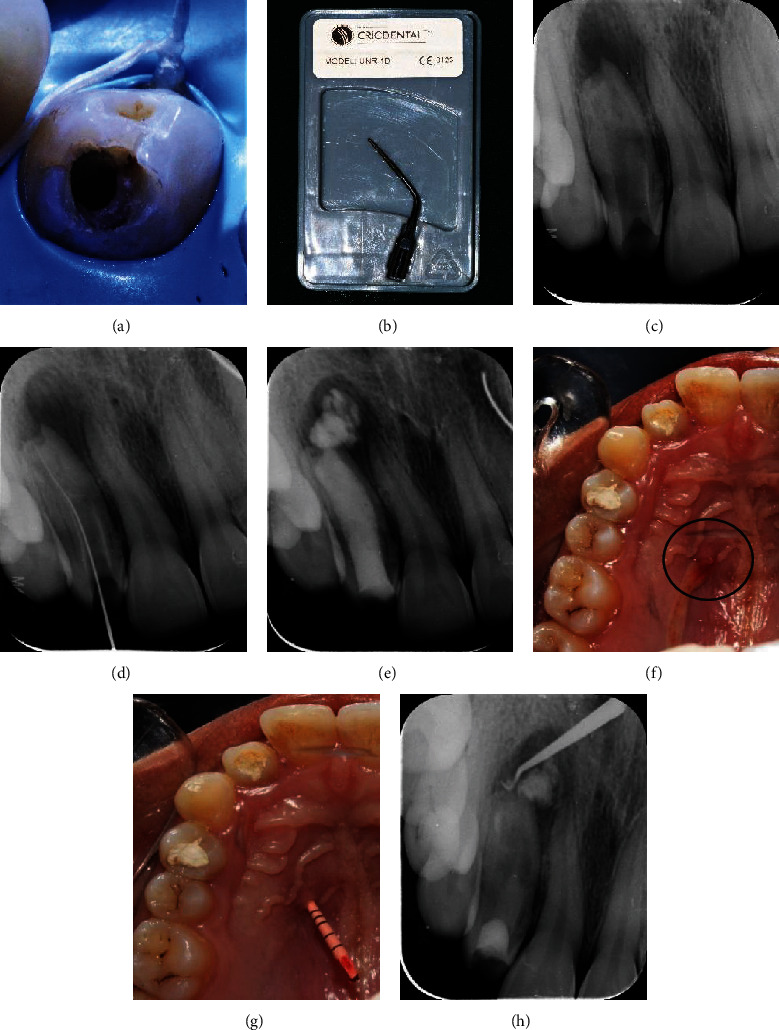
(a) Access was gained using round diamond bur. (b, c) Diamond-coated ultrasonic used to remove the invaginatus (d) working length. (e) Calcium hydroxide paste injected in the canal, leading to accidental extrusion. (f) Two weeks recall showing draining sinus on the palatal aspect. (g, h) GP tracing done after irrigation and replacement of calcium hydroxide dressing to powder and liquid form.
